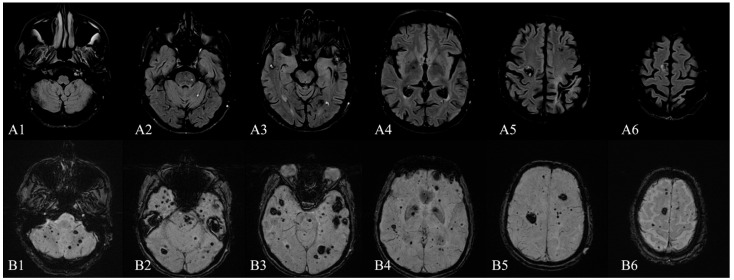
Brain MRI (1.5 T)—December 2015: (A1–A6) axial FLAIR sequence and (B1–B6) axial SWI sequence (TE 40.00 ms) showing multiple infra- and supratentorial, bilateral, cavernous malformations (lesions in the order of tens), without recent signs of bleeding. The characteristic MRI appearance of CCMs consists of well-circumscribed “popcorn-like” lesions of different sizes with a mixed-signal intensity core and a hypointense peripheral rim of hemosiderin conferring a “blooming” effect [1,2,3]. This heterogeneous appearance is due to the presence of blood products in various stages of degradation [4]. Cavernous malformations are present in 0.3–0.5% of the general population, although some postmortem studies suggest that this number could be higher, up to 4% [1,5]. In most cases, they occur in sporadic forms (80%), usually with solitary lesions, but familial cases have also been reported. Familial cases usually present with multiple, congenital, and acquired lesions, in various locations: cerebral, spinal, retinal, and cutaneous [6,7,8]. Topographically, the majority of CNS cavernous malformations have a supratentorial localization (76%), followed by infra-tentorial regions (19%), the spinal involvement being seldomly encountered (5%) [9]. Furthermore, concomitant lesions at all of the three levels are exceptionally rare and they are a particularity of the familial forms of cavernous malformations [10,11]. Clinically, the majority of cerebral cavernous malformations (CCMs) become symptomatic between the second to fifth decades with findings such as seizures (23–50% of cases), headaches (6–52%), focal neurological deficits (20–45%) or hemorrhages (9–56%) [2,9,12]. Up to 40% of patients with such malformations are asymptomatic [12]. Imaging-wise, T2-weighted, gradient-echo MR (T2-GRE), high-field MR and especially susceptibility-weighted (SWI) MR sequences are the most sensitive techniques for detecting CCMs [1,13]. Here, we present the case of a 65-year-old man diagnosed in 2015 in our clinic with multiple supra- and infratentorial CCMs (Figure 1), whom we were following for secondary epilepsy with rare seizures with focal onset, impaired awareness, and secondary generalization. He used to take carbamazepine, but he had discontinued the treatment for the last three years despite our recommendations. More recently, he had been receiving a direct-acting oral anticoagulant indicated by the cardiologist as primary prophylaxis of cardioembolic stroke from atrial fibrillation with a CHA_2_DS_2_-VASc score of 2. Recently, in 2021, the patient was re-admitted into our clinic, this time for postural instability and motor deficit with gait impairment, with the onset three days prior. The initial neurologic examination showed temporo-spatial disorientation, hypophonia, right-side central facial paresis, asymmetric tetraparesis (force grading on the MRC scale of 4/5 in the upper limbs and 3/5 in the lower limbs), with the right side slightly more affected, upper limbs dysmetria, brisk deep tendon reflexes with Babinski sign, abnormal ataxic gait, which was possible for only a few steps with bilateral support. We also noted a significant cognitive decline from MMSE of 23 points in 2015 (mainly due to attention, orientation, and executive functions deficits) to severe dementia with a MMSE of 8 points in 2021, when the patient presented significantly slowed behavioral reaction time with failure to initiate Trail Making Test part B, reasoning difficulties and forgetfulness.
